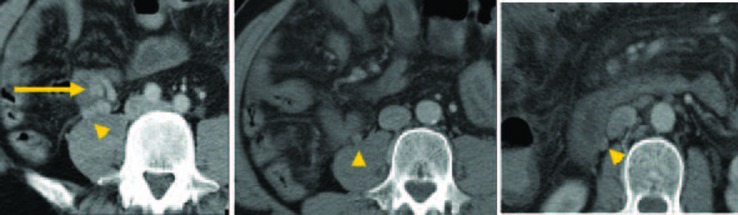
Computed tomography images of a vessel in the ileum (arrow) and its connection to the right testicular vein (arrowhead).
